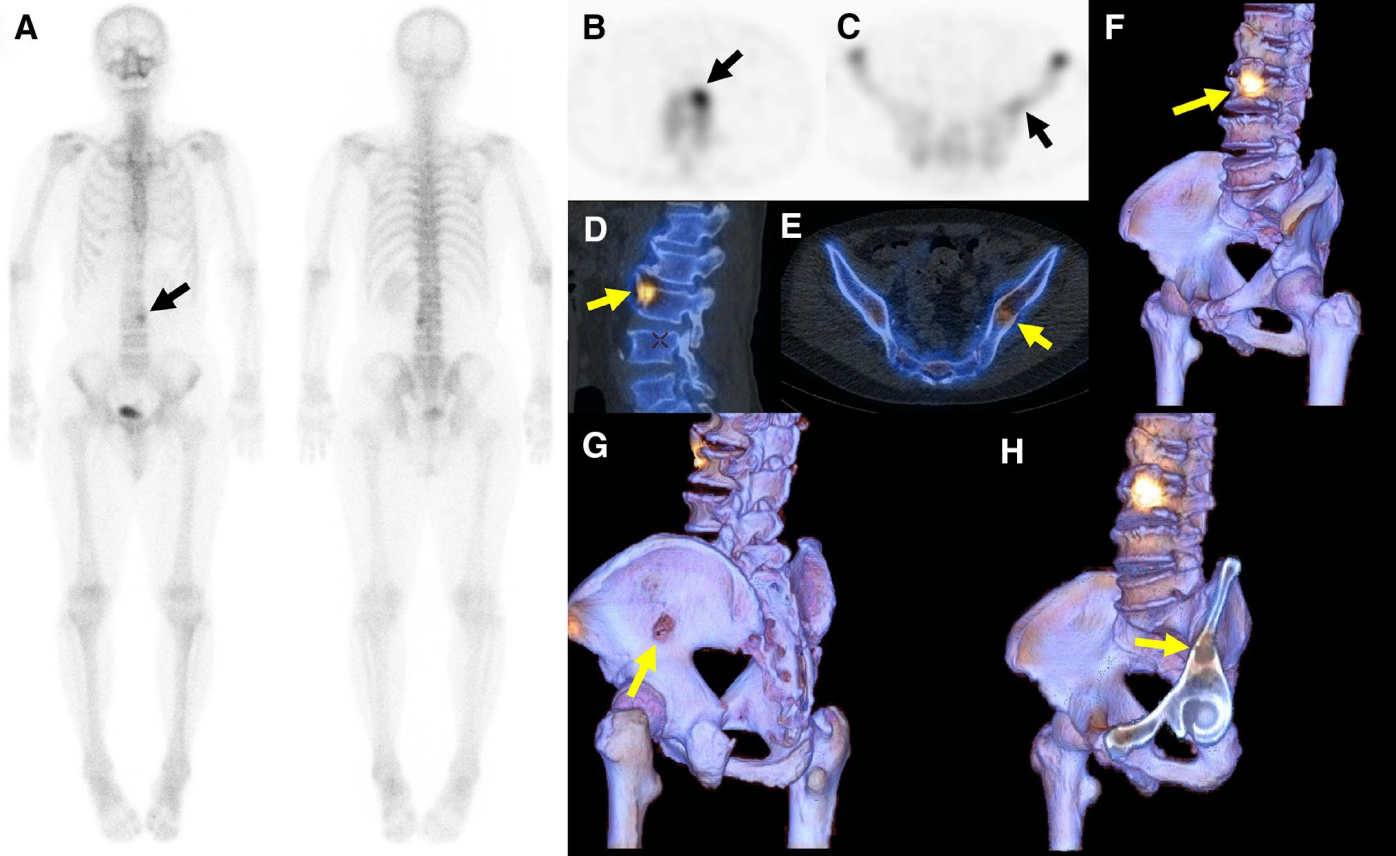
Whole-body scintigraphy (a), SPECT (b and c), 2D SPECT/CT fusion (d and e), and image data projection of bone SPECT onto 3D volume-rendered CT images without (f and g) or with clip-plane editing (h) in a renal cancer patient with co-existence of an osteolytic metastasis in the left iliac bone and a hypermetabolic osteophyte in the third lumbar vertebra. The iliac lesion was barely visible in whole-body scintigraphy (a) and SPECT showed faint uptake (c), suggesting benign nature rather than metastasis. Osteolytic change is clearly seen in 2D SPECT/CT (e) and our 3D SPECT/CT shows a hole at the slightly hypermetabolic site (g). Clip-plane method allows the visualization of an osteolytic lesion with minimal pathological fracture (h)
